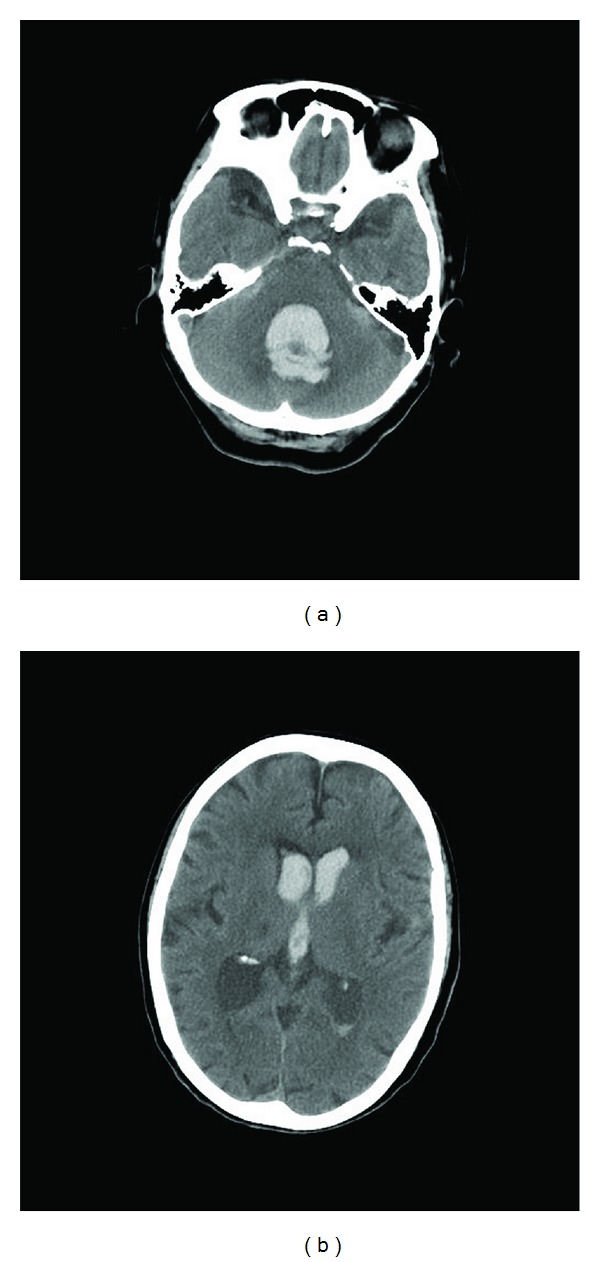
CT scan of the head was performed on admission day. Cerebellar hemorrhage in vermis (a) and intraventricular bleeding (b) were observed.
